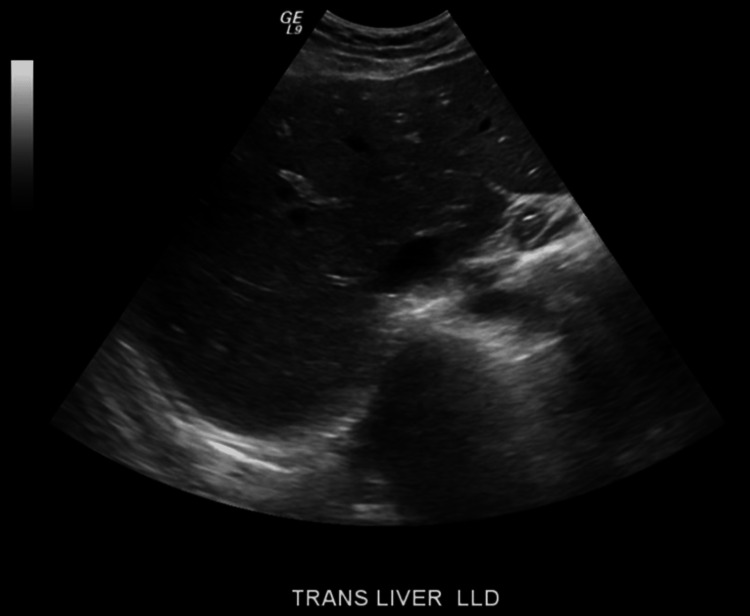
Ultrasound of the liver showing a normal liver architecture
